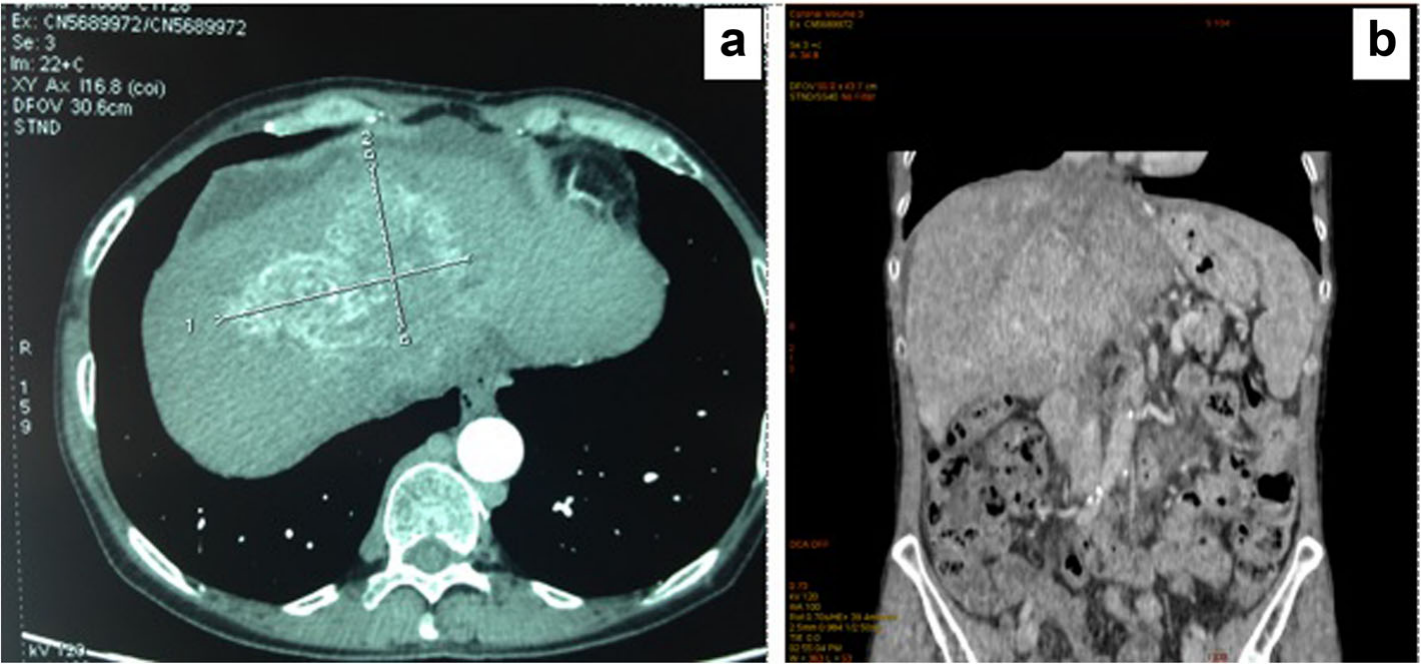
Pre-Transplant CT-scans presenting: a Computed Tomography of a 110 × 75 × 70 mm hepatic alveolar echinoccocal cyst located in segments III–VIII; b Hepatic vein thrombosis – Budd-Chiari Syndrome
